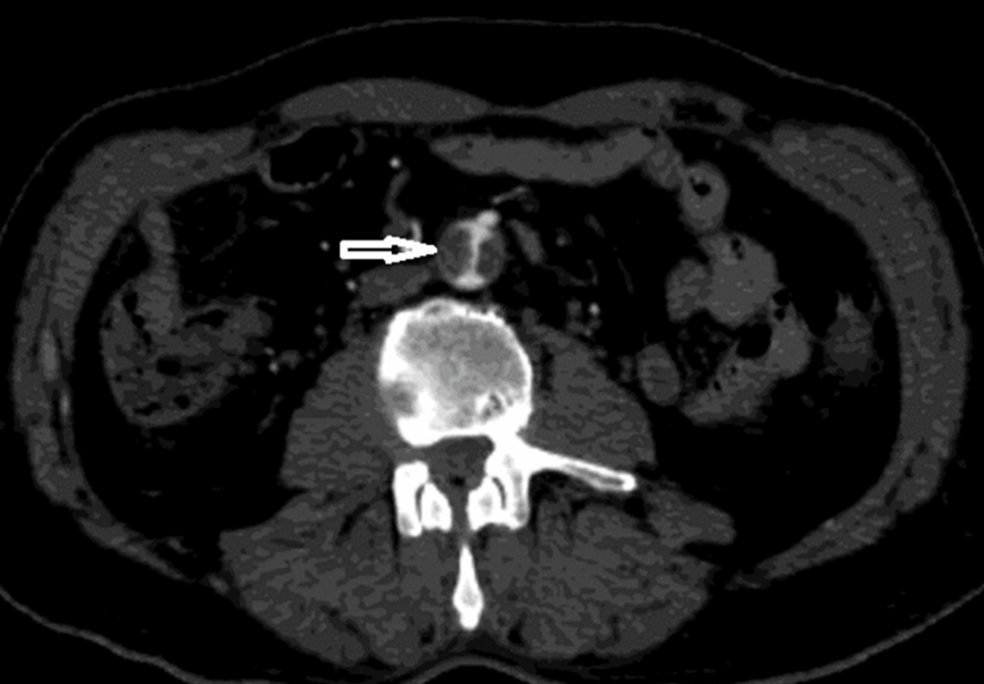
CT angiography on axial view revealing an intraluminal abdominal aorta thrombus (white arrow)
